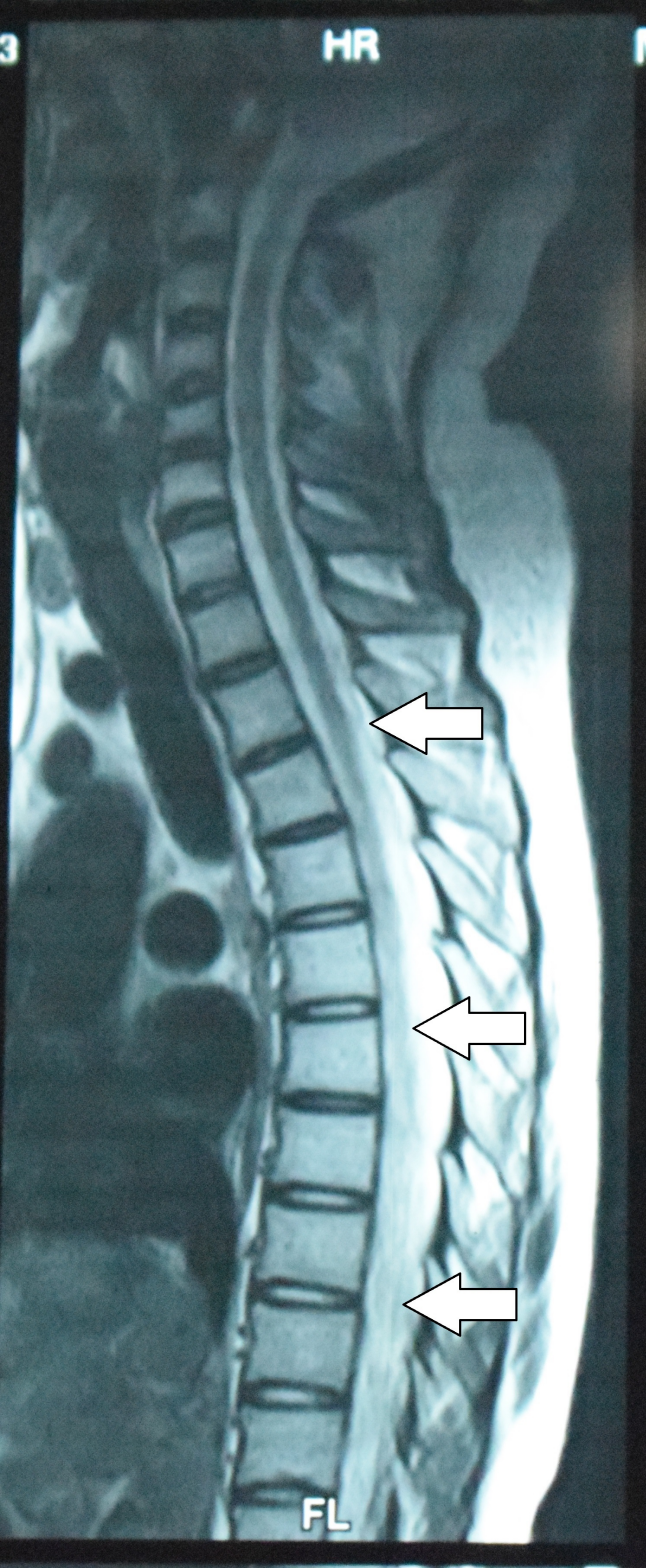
Spinal MRI (saggital) of the dorsal column. T-2 weighted image shows hyper-intense signals between T5-L1 (arrows). MRI: Magnetic resonance imaging.
